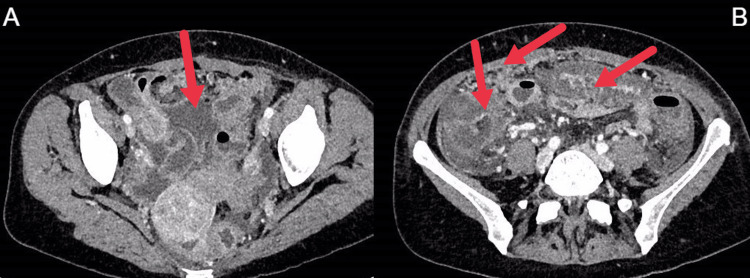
High-resolution abdominal CT images showing ascites (A, arrow) and an enlarged right ovary, omental infiltration, and bowel wall thickening with marked submucosal edema (B, arrows). CT: computed tomography
